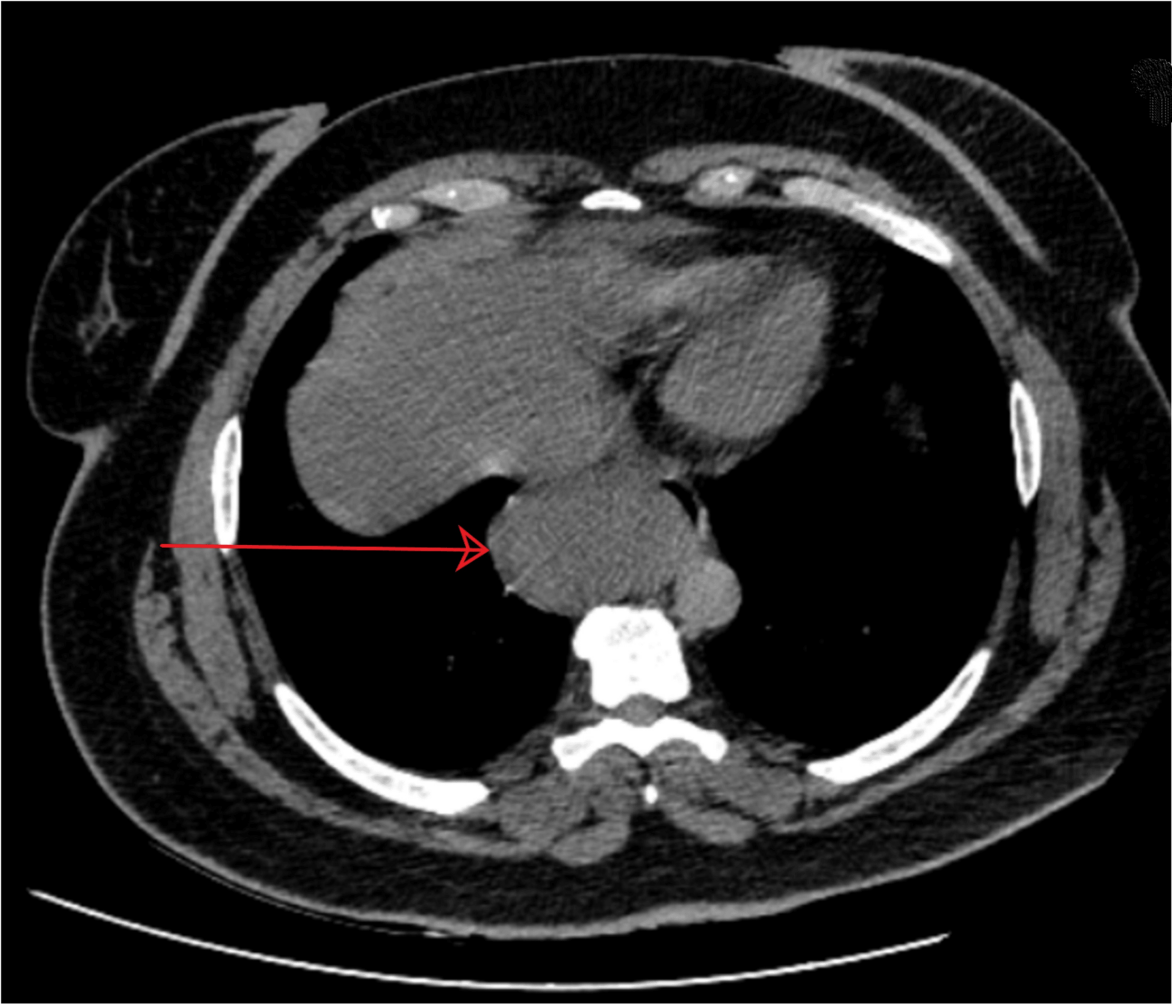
Submucosal tumor of the lower esophagus extending to the right part of the mediastinum Axial plane of thoracic computed tomography (non-contrast)
